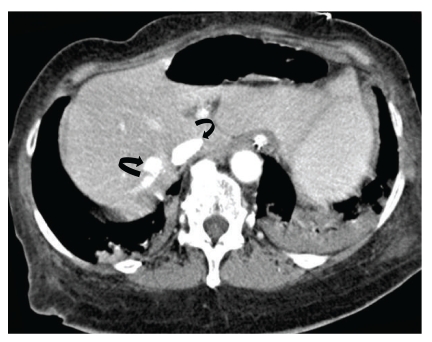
CT image of the liver shows normal contrast enhancement in the inferior vena cava and hepatic vein (*curved arrows*).
